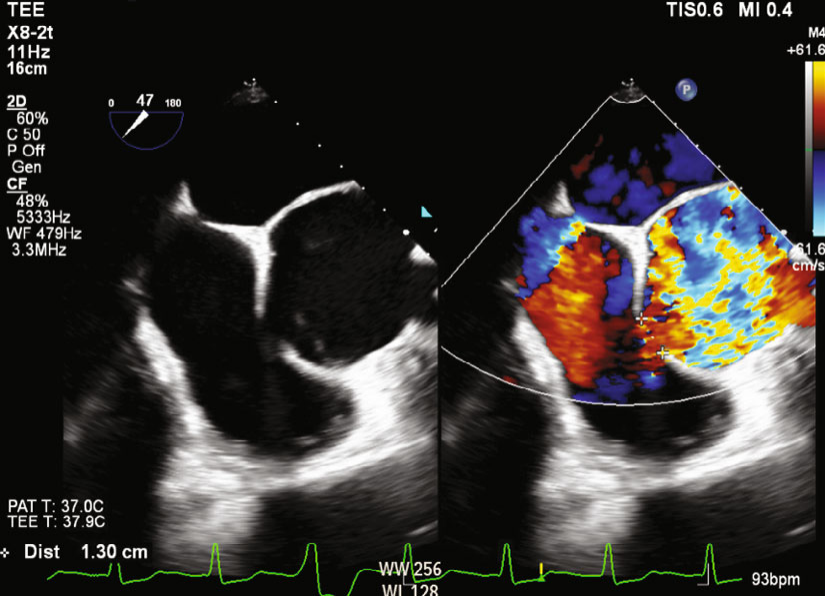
Diagnostic transesophageal echocardiogram. Demonstration of a 6.5-cm aortic root with a dissection flap into the wall of the right coronary cusp and rupture into the right atrium.
